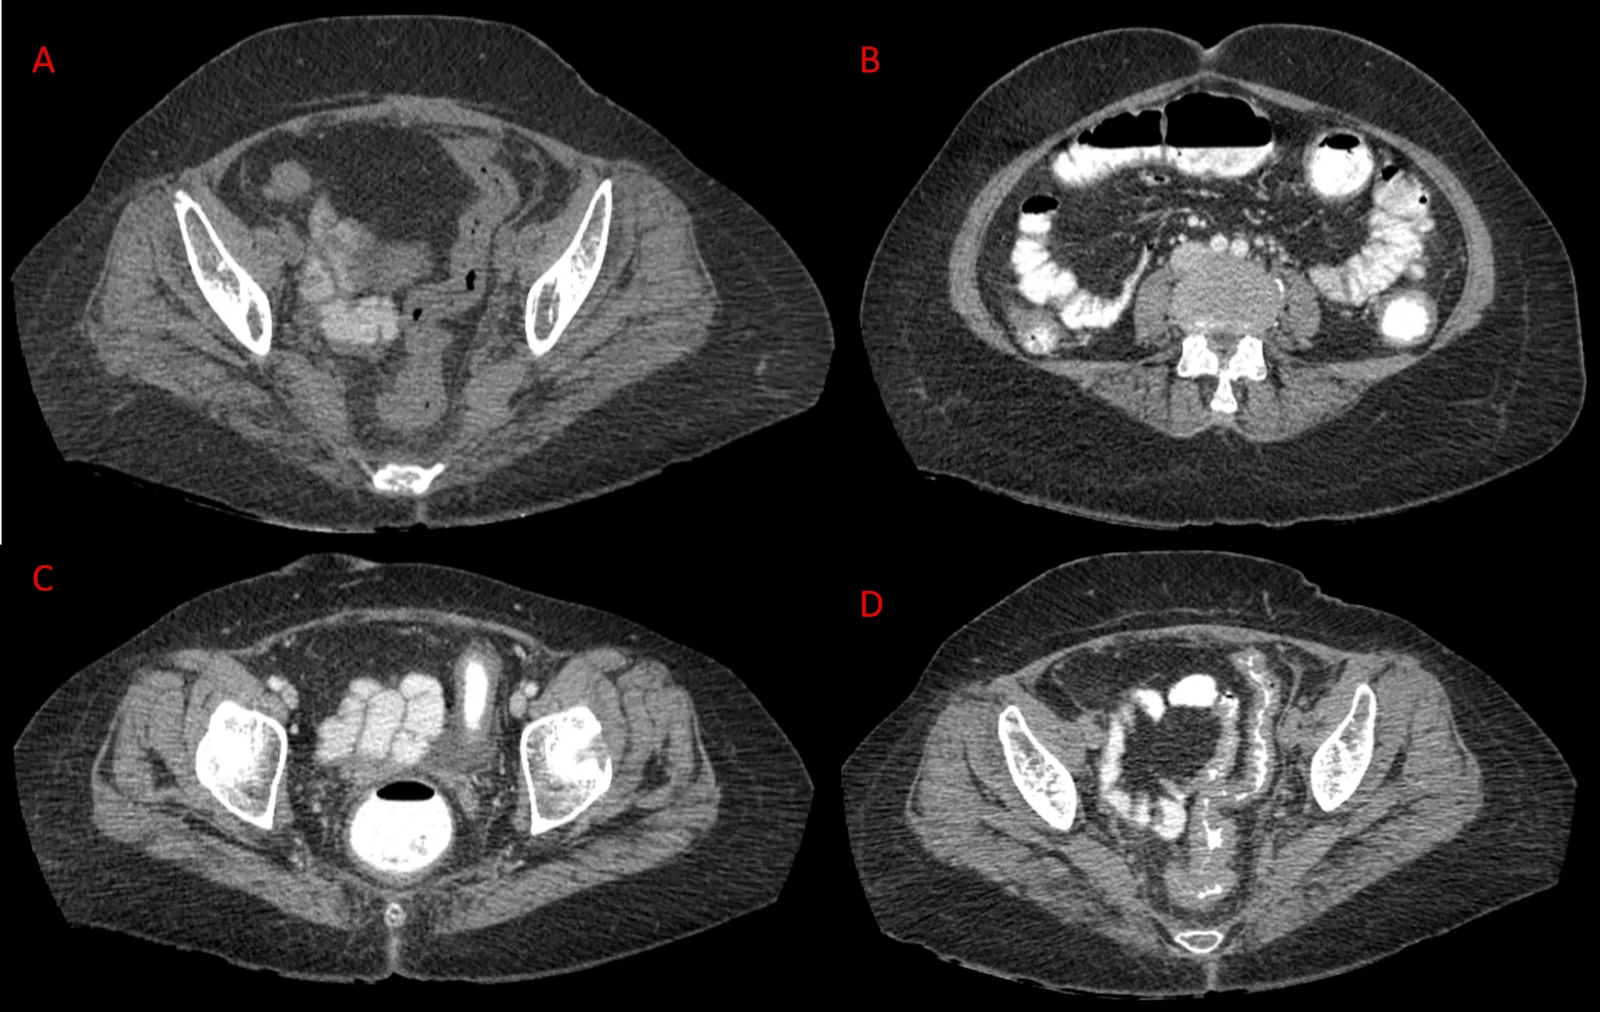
Contrast-enhanced computed tomography (CT) of the abdomen and pelvis (A-D) Diffuse mild circumferential wall thickening of the rectum, sigmoid colon, descending colon, and distal transverse colon, with mild pericolonic fat stranding.
